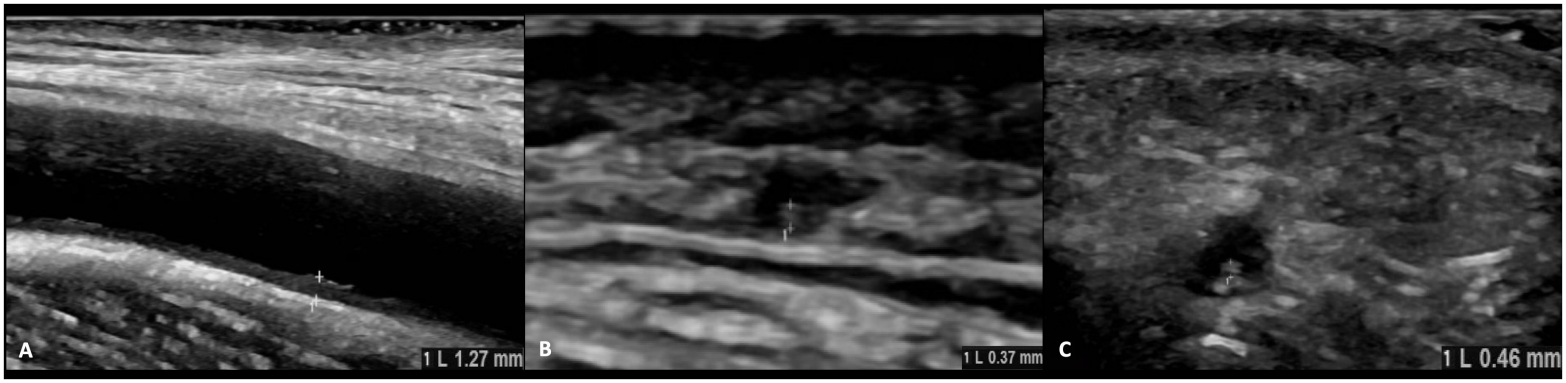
Vascular ultrasound and pathological intima-media thickness. This figure presents the outcomes of a detailed ultrasound evaluation of the superficial temporal arteries along with their branches, and the facial, axillary, brachial, subclavian, and carotid arteries, displaying those with pathological intima-media thickness. The right axillary artery (1.23 mm), right frontal lobe of the temporal artery (0.37 mm), and right facial artery (0.46 mm) surpass the established threshold values with no changes in the extent of vascular involvement in comparison to previous examinations. (A) right axillary artery, (B) right ramus frontalis, (C) right facial artery.
